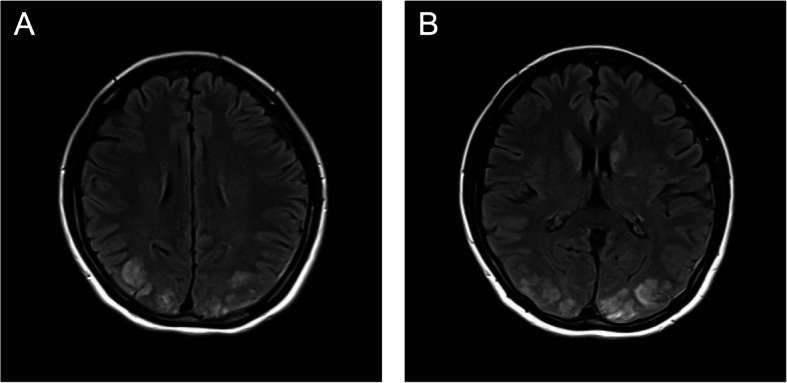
Magnetic resonance imaging (MRI) findings. Axial FLAIR images show hyperintensities in the parietal lobes (A) and occipital lobes (B) bilaterally. FLAIR: fluid-attenuated inversion recovery
